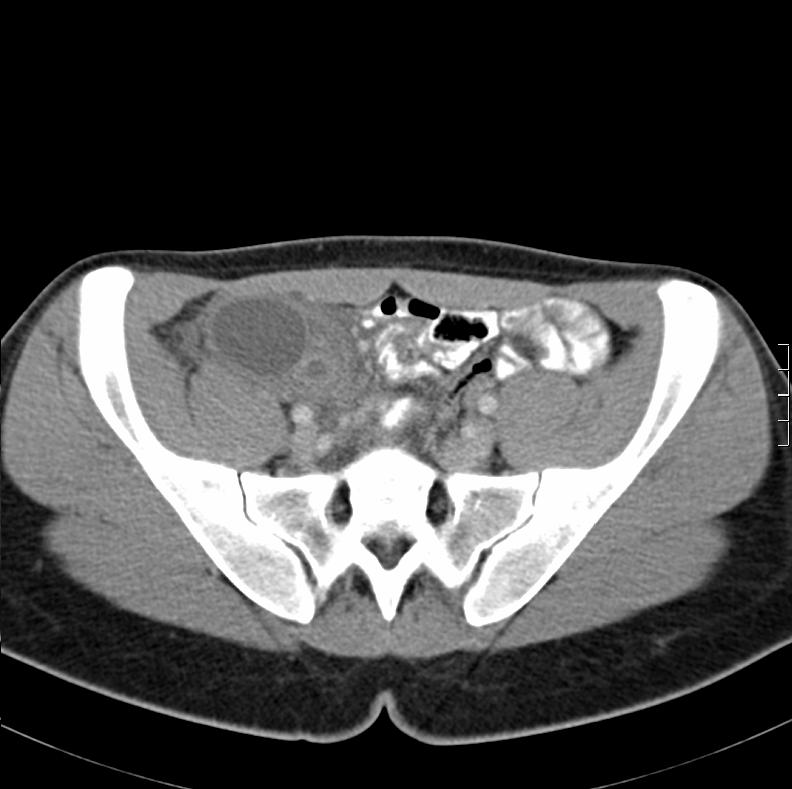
Axial CT image showing a loculated cystic mass in the right pelvis which appears to contain the tip of the appendix.
